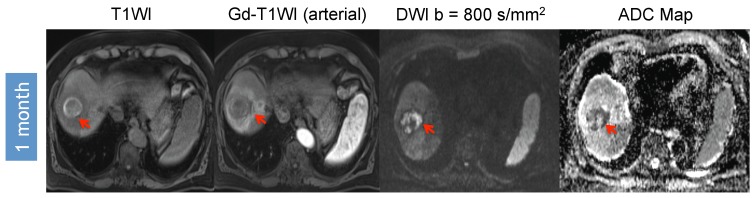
Hemorrhage causes “pseudo-restriction” on DWI. 62-year-old man with segment VIII HCC (arrow) without macrovascular invasion or extra-hepatic disease who underwent radioembolization segmentectomy therapy. There is no Gd-enhancement on one month post-procedural MRI. However, there is significant hyperintensity on pre-contrast images. Although the absence of enhancement correlates with absent diffusion restriction, the presence of hemorrhage creates “pseudo-restriction” without the presence of viable tumor. This pitfall has to be recognized.
